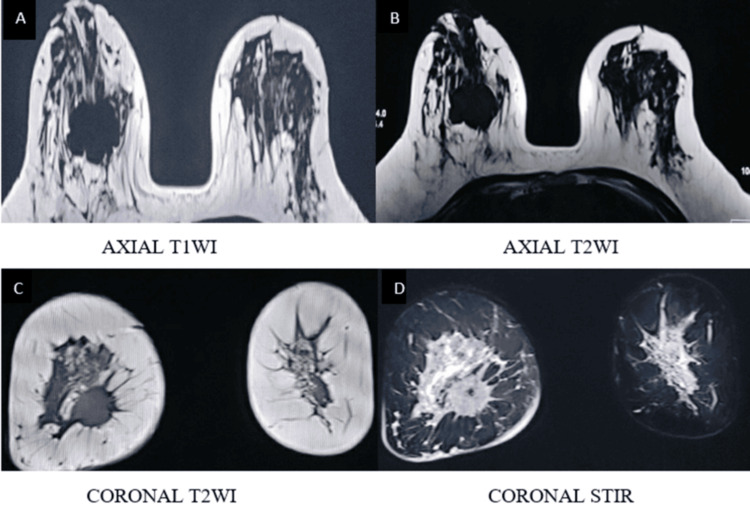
A spiculated mass lesion in the lower inner quadrant of the right breast appears isointense on T1WI and T2WI (A, B, C) and hyperintense on STIR images (D) T1WI: T1-weighted image; T2WI: T2-weighted image; STIR: short TI inversion recovery
